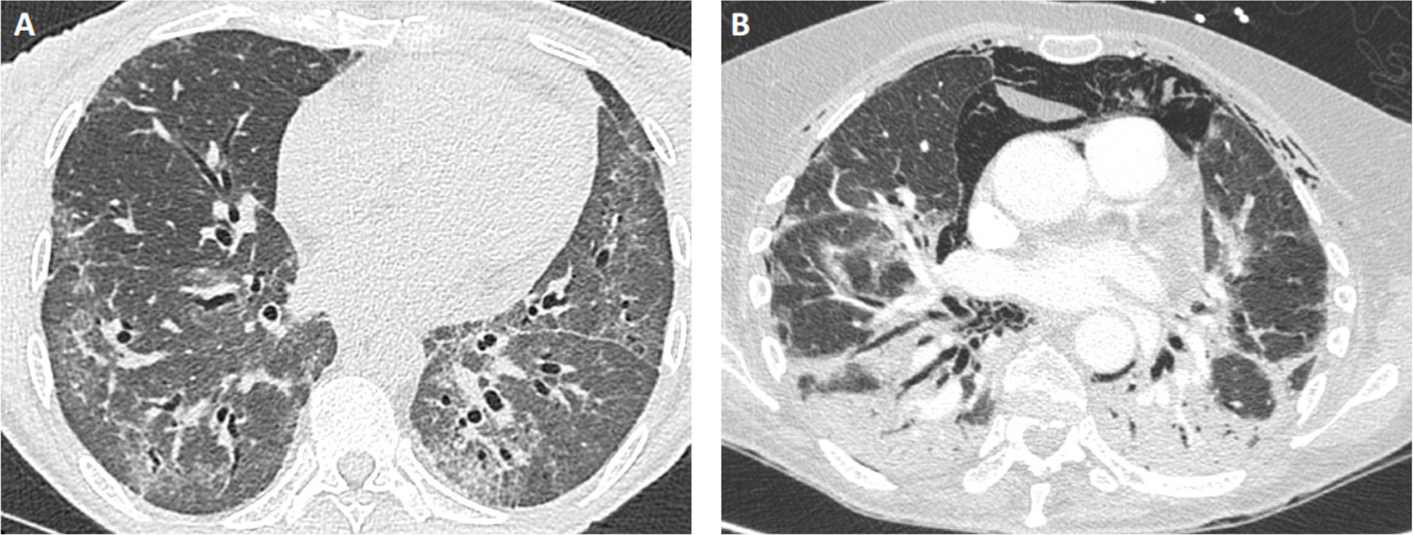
Examples of lung CT findings in patients with IM-related ILD. (A) NSIP pattern in a patient with PL12+ antisynthetase syndrome: ground glass opacities with bilateral proximal bronchiectasis. (B) OP pattern in a patient with MDA5+ dermatomyositis: extensive parenchymal consolidation and pneumomediastinum.
